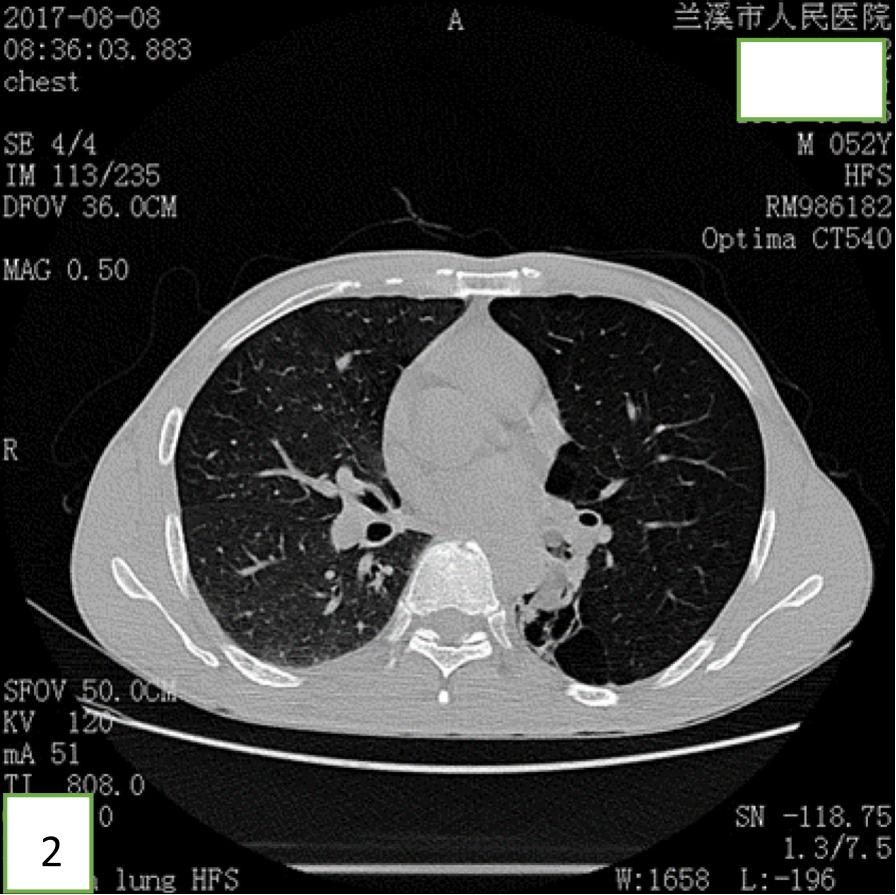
CT suggesting low-density mass obstructing the left lower lobe, the CT value is -98HU
